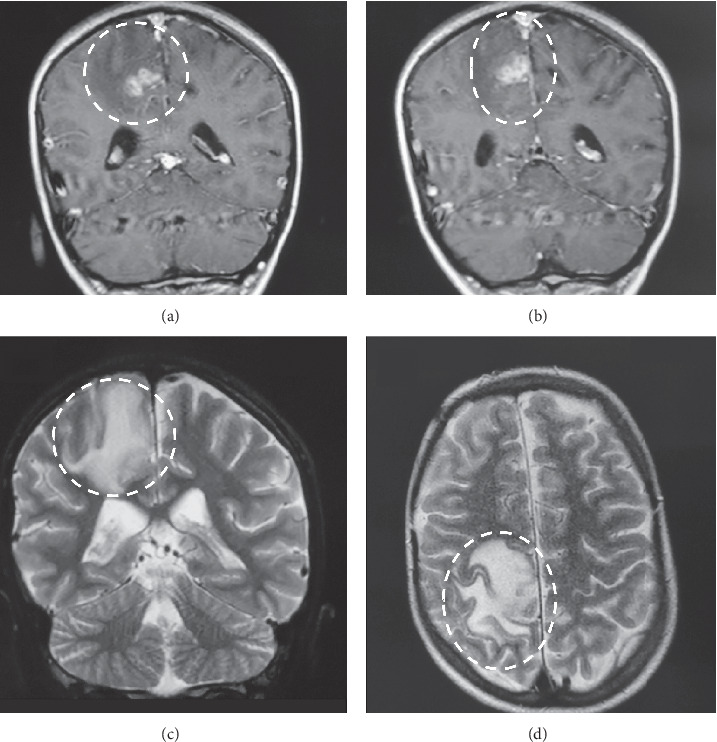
Brain magnetic resonance imaging (MRI): 2.2 × 2.0 cm ring-enhancing parasagittal lesion in the right parietal lobe.
